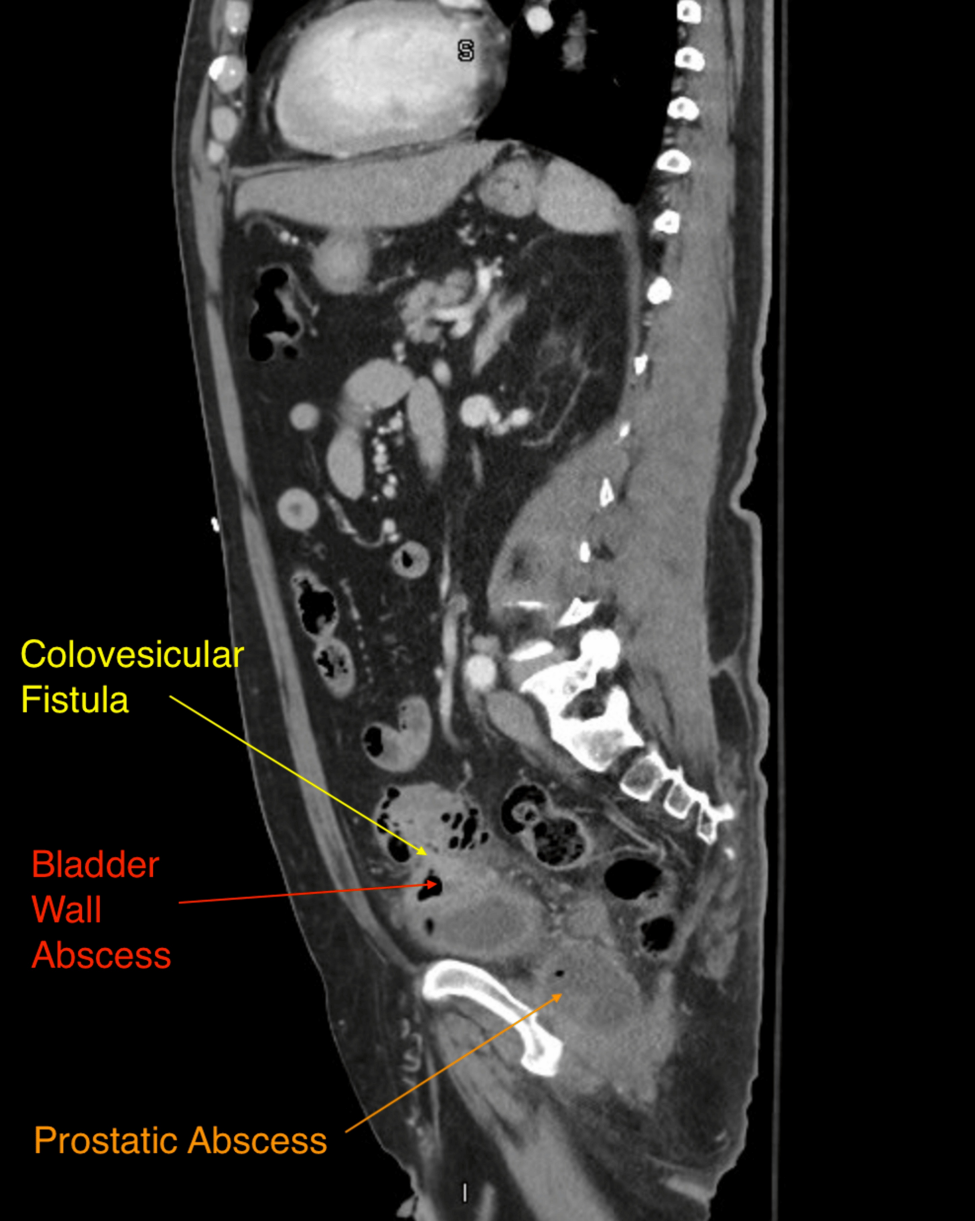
CT (sagittal view) showing colovesicular fistula, bladder abscess, and prostatic abscess
